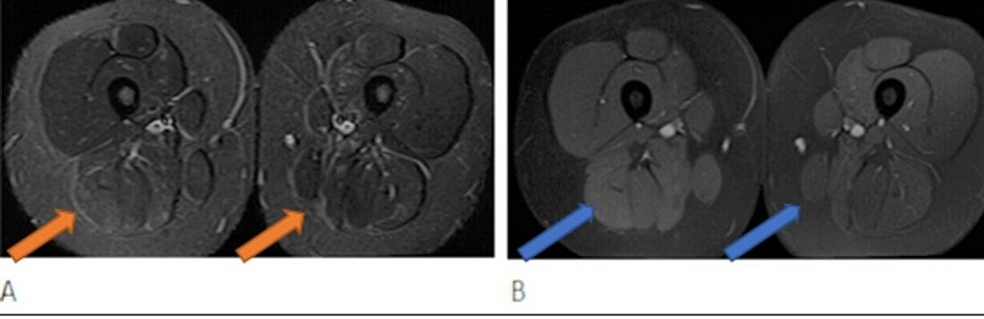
MR images of the lower thighs. Axial MR images of the lower thighs in 2020. A: STIR image demonstrates hyperintense signal in the muscles of posterior compartment, like the study from 2017. B: Post contrast T1 weighted image demonstrates subtle enhancement within the areas of signal abnormality of the muscles, like the prior study from 2017 (see Figure 2).
